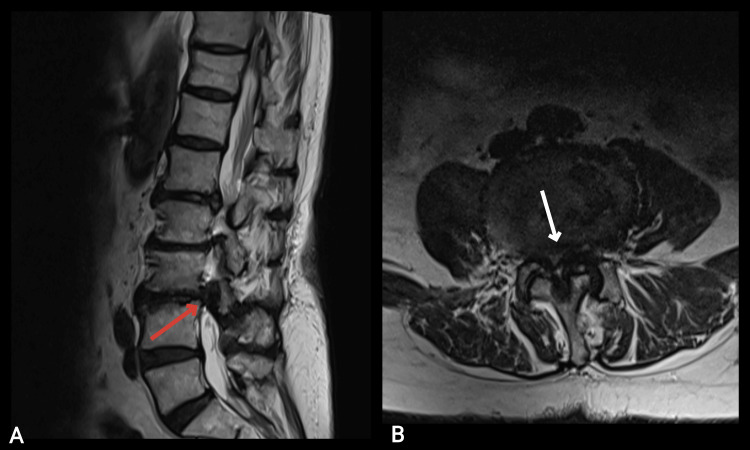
Preoperative MRI of an 85-year-old woman showing high-grade spinal stenosis in the sagittal plane (red arrow) and axial plane (white arrow) at the L3-L4 level. A: T2 sagittal; B: T2 axial.
